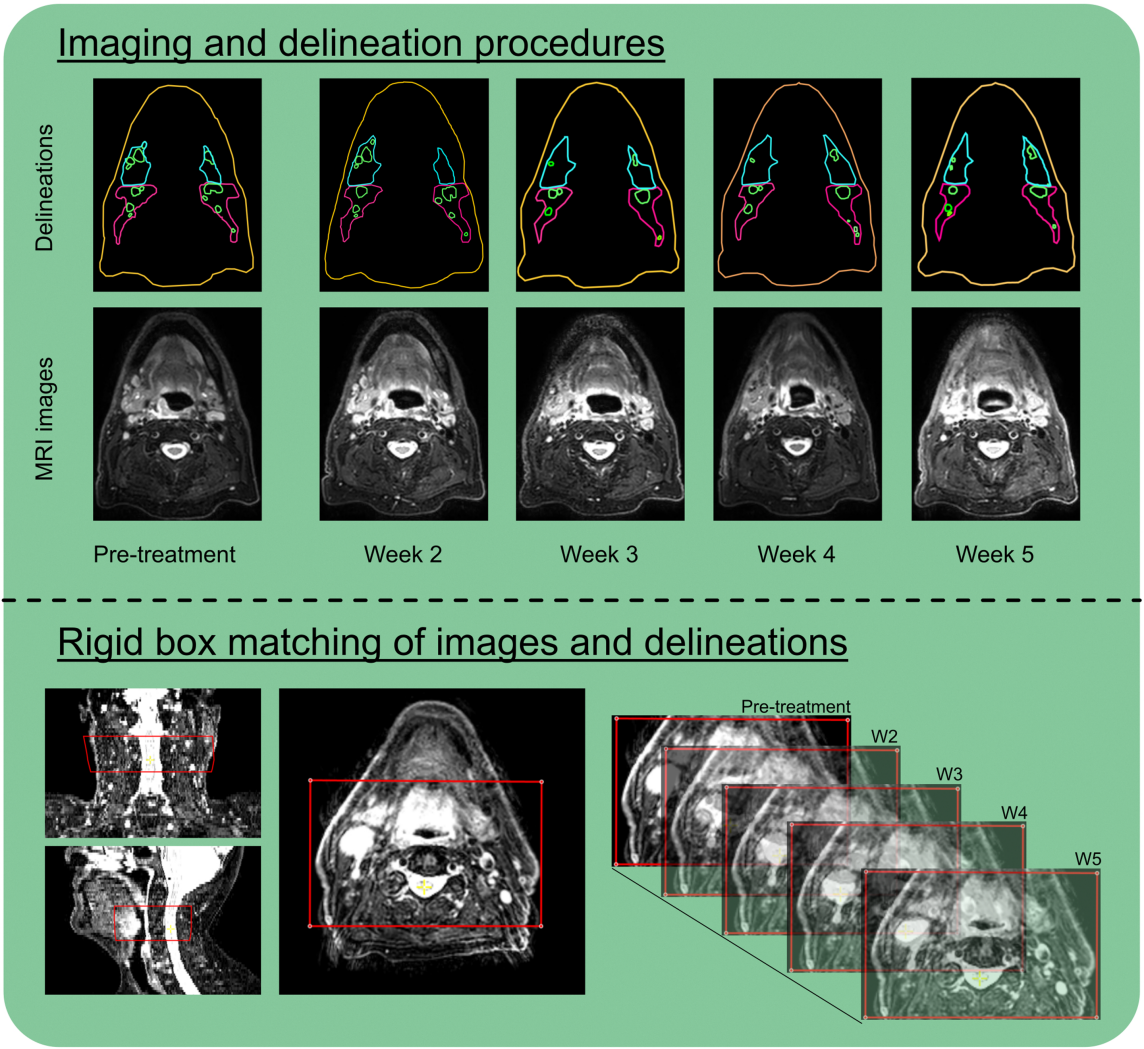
For this study per patient in total 5 MRI scans were obtained in which elective lymph nodes and lymph nodes levels were delineated (top). All scans and delineations were rigidly matched using a box around spine and lymph node levels at the level of the tumor (bottom).
